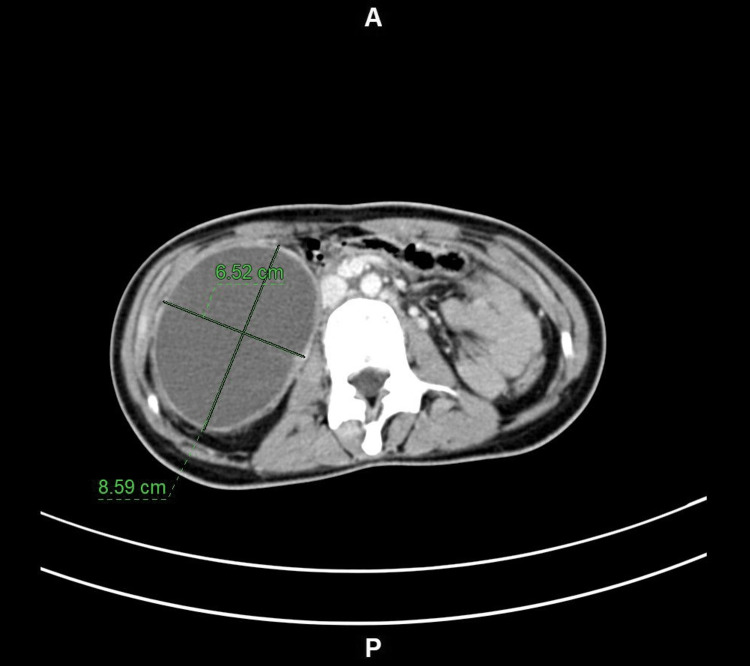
Computed tomography view of the mesenteric cyst
